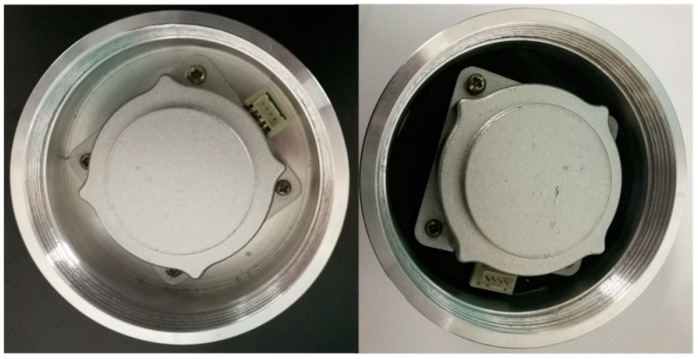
Ultrasonic motor with (right) and without (left) the protection of rubber.
